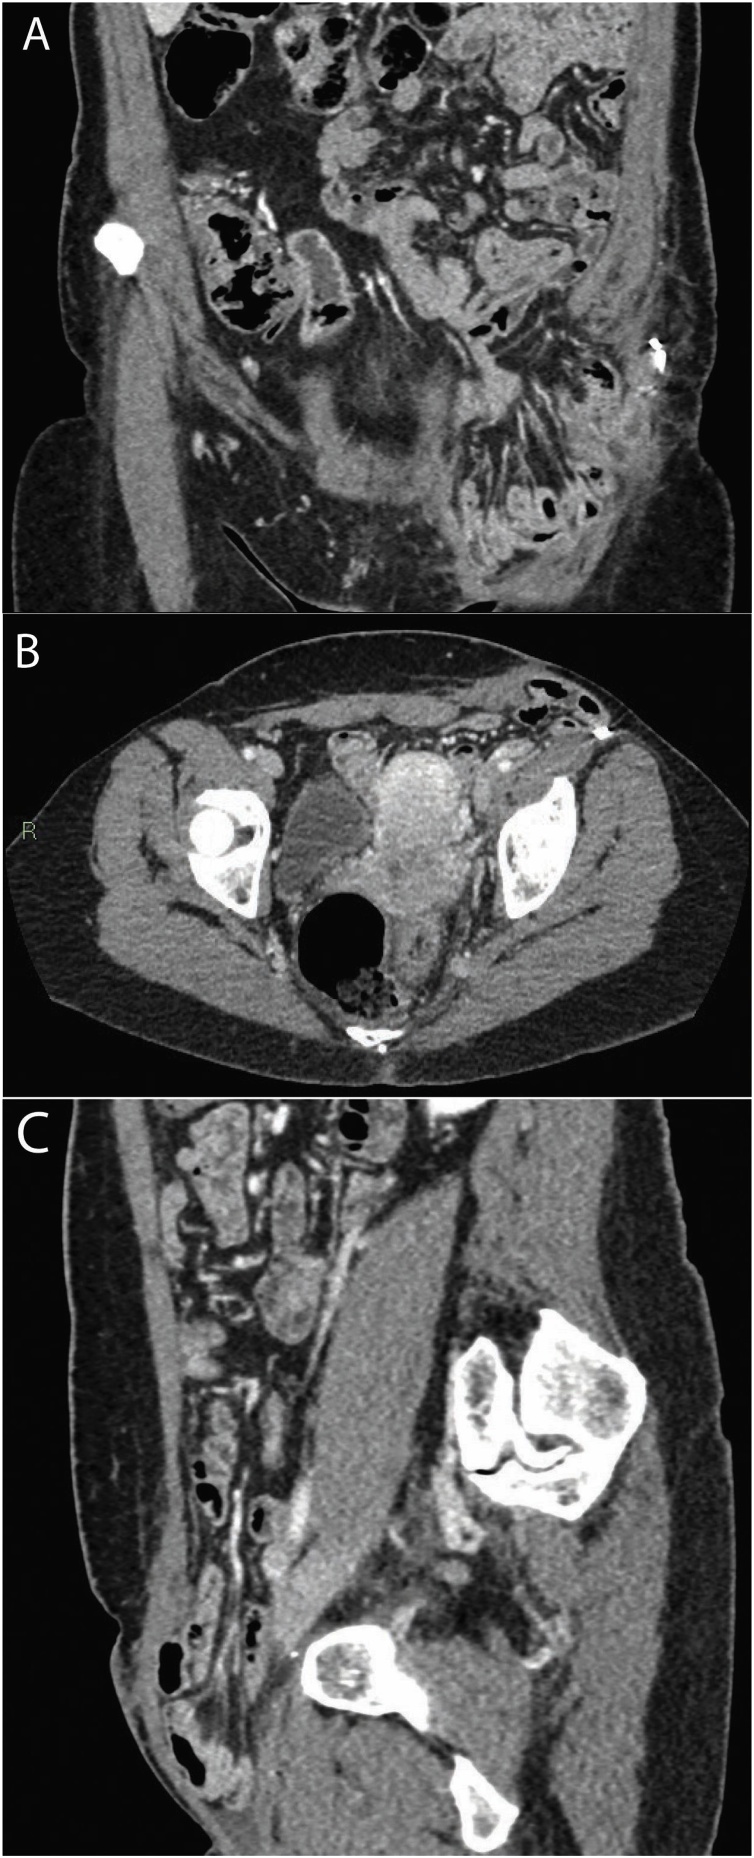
CT scan demonstrating a large incisional inguinal hernia, with clustered small bowel loops descending into the groin area. Coronal (A), transversal (B) and sagittal (C) sections.
